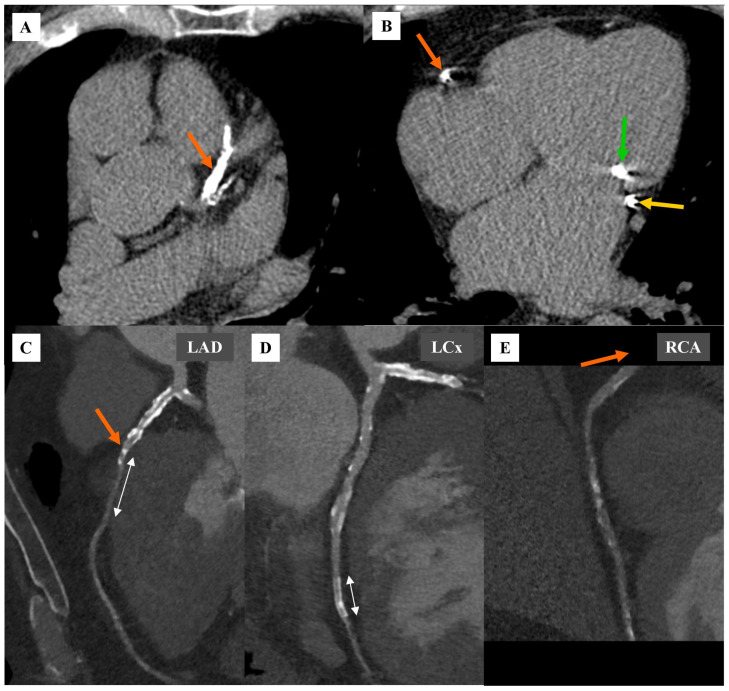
An 80-year-old Caucasian man with chronic coronary artery disease, who had undergone percutaneous coronary intervention (PCI) of the middle segment of the left circumflex artery (LCx) with drug-eluting stent (DES) implantation, was referred to the computed tomography (CT) department for coronary computed tomography angiography (CCTA) due to difficulty visualizing the right coronary artery (RCA) during invasive coronary angiography (ICA). The CCTA examination was performed using a 384-slice dual-source SOMATOM Force computed tomography scanner (Siemens Healthcare, Erlangen, Germany) using a standard protocol (described in other publication by the authors [1]). After coronary CTA, the patient is scheduled for further diagnostics and treatment at the treating center. (A) Native phase. Axial view. Coronary artery calcium score assessment. Massive calcifications in the left anterior descending (LAD) are marked with an orange arrow. (B) Native phase. Axial view. Coronary artery calcium score assessment. Massive calcifications in the LCx are marked with a yellow arrow, in the RCA with an orange arrow, and in the mitral valve with a green arrow. (C) Angiographic phase. Curved multiplanar reforming (cMPR) reconstruction. LAD. Multiple, predominantly calcified atherosclerotic plaques causing coronary stenosis. The orange arrow indicates stenosis of the mid-segment of the LAD artery, estimated by CT to be greater than 70%. The white marker indicates a muscular bridge in the mid-segment of the LAD artery, approximately 2.5 cm long. (D) Angiographic phase. Curved multiplanar reforming (cMPR) reconstruction. LCx. Multiple, predominantly calcified atherosclerotic plaques. The white marker indicates a stent in the mid-segment of the LCx artery, approximately 1.5 cm long and 0.3 cm in diameter. The stent diameter in the LCx artery was borderline for assessing its patency. No clear signs of stent restenosis were observed. (E) Angiographic phase. Curved multiplanar reforming (cMPR) reconstruction. RCA. The orange arrow indicates the lack of reconstruction of the proximal segment of the RCA, resulting from arterial occlusion. The middle and distal segments of the RCA, presumably filled with collateral circulation, have significantly weaker contrast, and contain numerous predominantly calcified atherosclerotic plaques, causing coronary stenosis. Assessment of the degree of stenosis is unreliable due to poor artery contrast.
